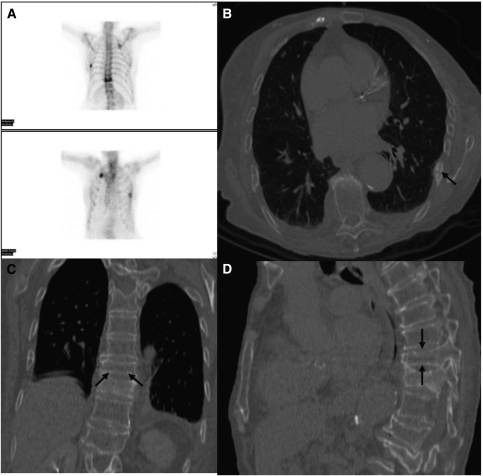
False positive. Bone scintigram (A) and CT chest examination (B, C, D) in a patient with stage IV breast cancer. The bone scan was reported as showing hot spots in the T10 vertebrae, left posterior seventh and right anterior second ribs, consistent with metastases. The follow-up CT scan revealed these changes to be due to rib fractures (B), and degenerative disease (C, D) only.
